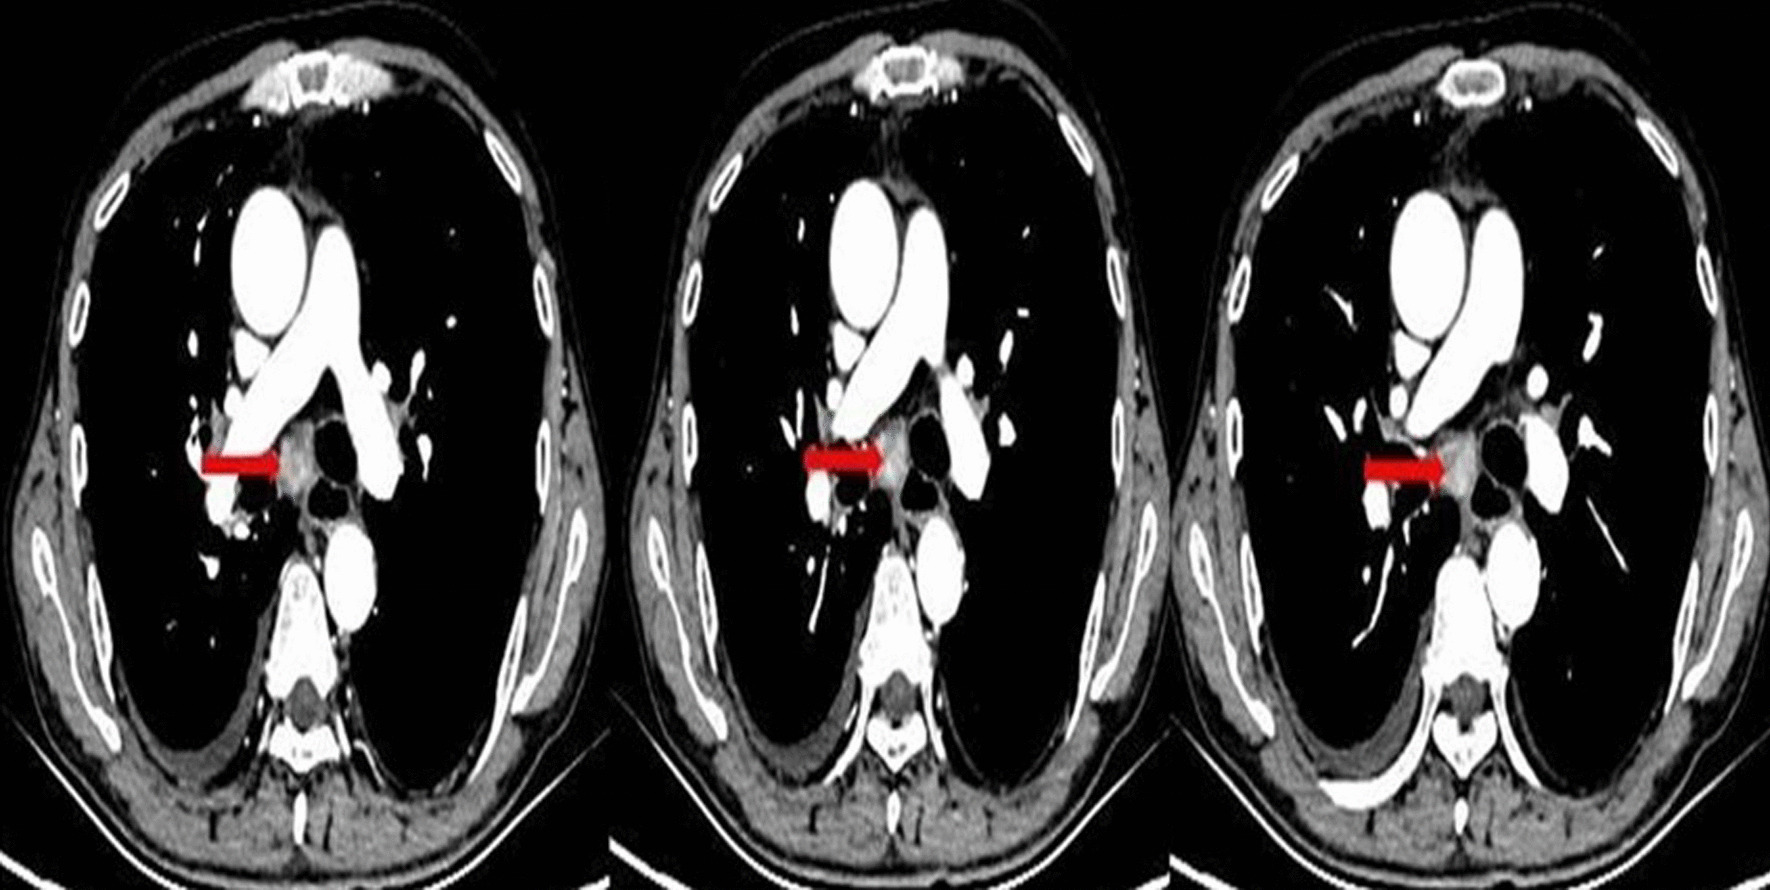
Enhanced CT revealed enlargement of the paraesophageal lymph nodes
